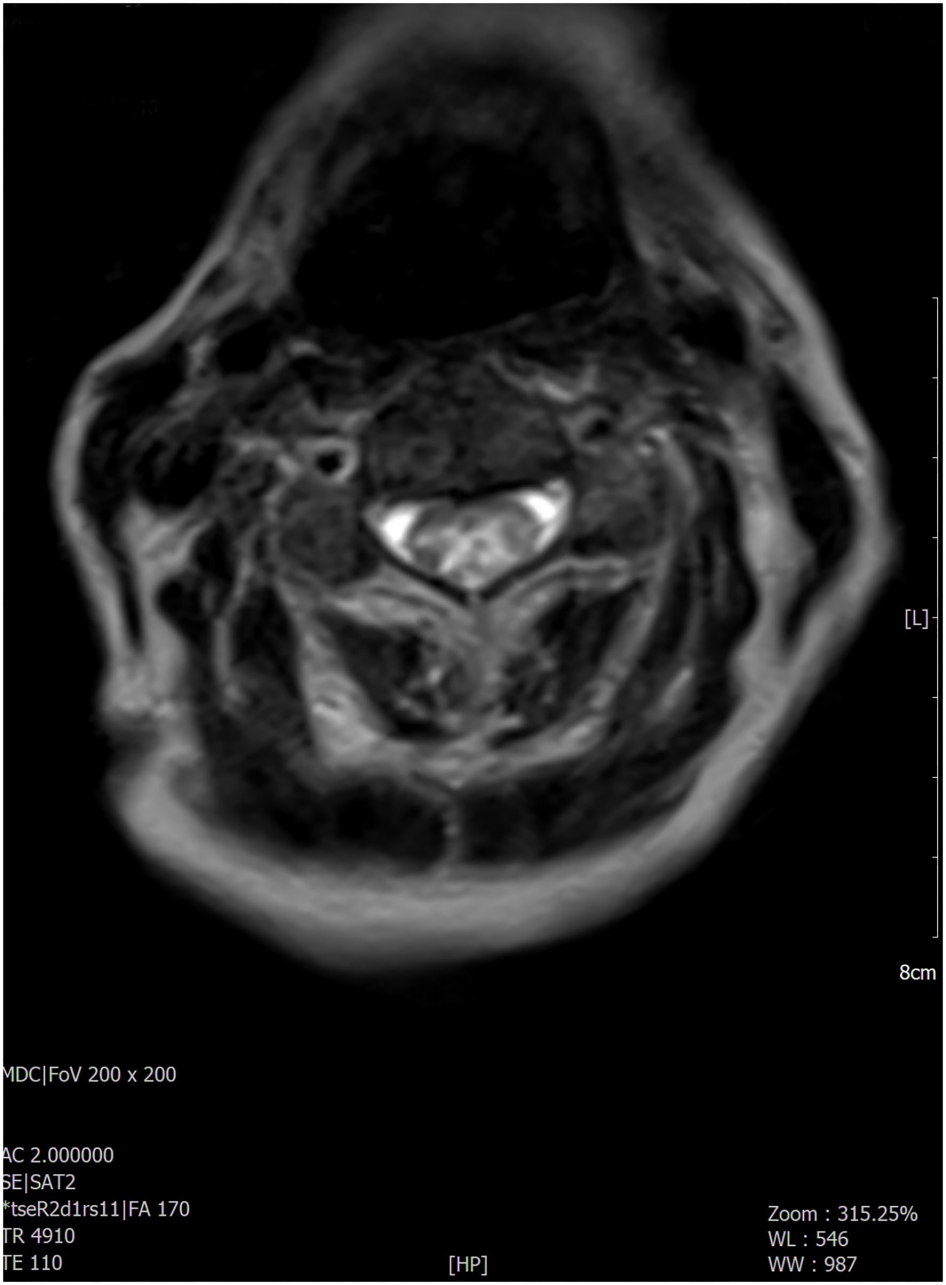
The most significant findings were found at the level of the cervico-dorsal cord with evidence, in the T2-weighted sequences, of extended signal hyperintensity which mainly concerned the central component and in particular the gray matter.
